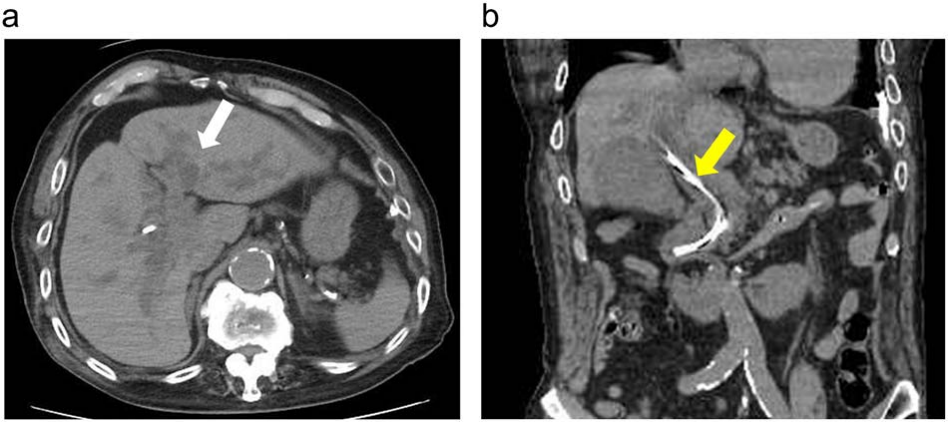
(a) Computed tomography showing dilated intrahepatic bile ducts (white arrow). (b) Computed tomography showing a bile duct stent (yellow arrow).
